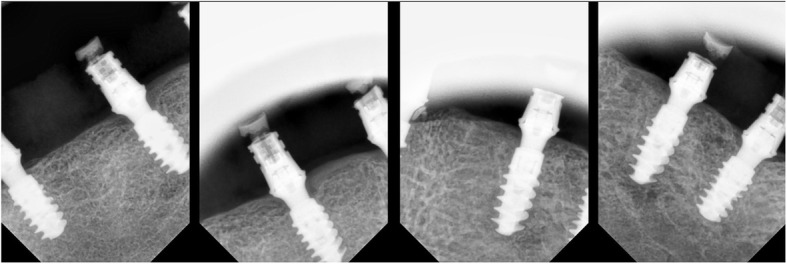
Periapical X-rays at 1 year
